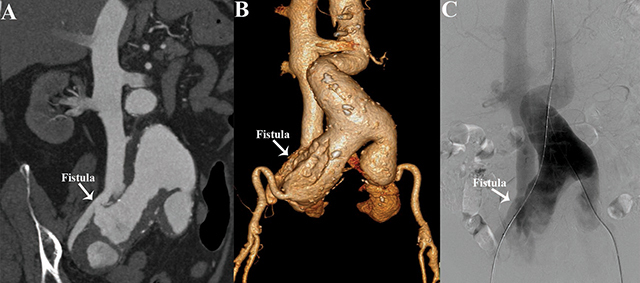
(A and B) Multiplanar reconstructions (MPR) and 3D volume rendered (3D-VR) CTA images show a large ruptured abdominal aortoiliac aneurysm causing an ilio-iliac arteriovenous fistula. (C) Abdominal aortography Abdominal aortography reveals ilio-iliac AVF with a fistula size of 8.5mm.
